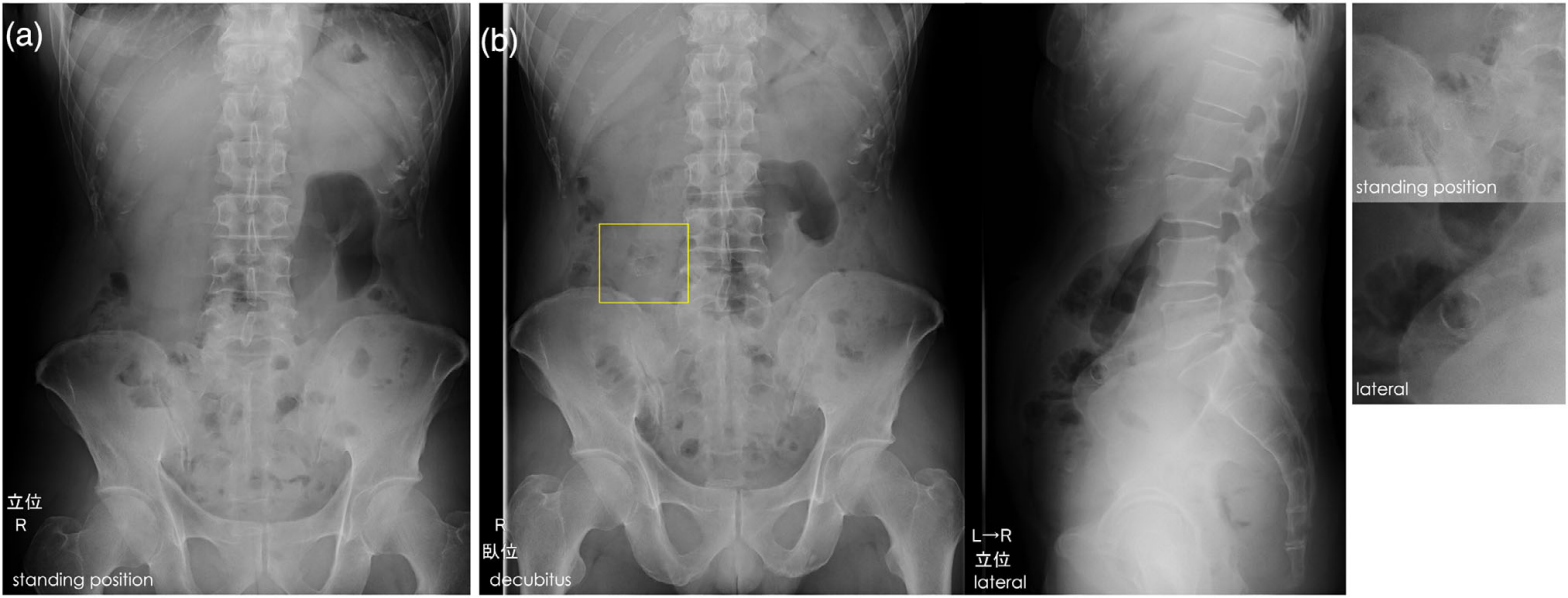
Plain abdominal X‐ray imaging. (a) Plain abdominal radiography (standing position) showed no patency capsule or shape deformity. (b) Plain abdominal radiographs (lateral and decubitus positions) showing the localization of the patency capsule (PC, in the lateral and decubitus views. The PC had already dissolved into a shell only (yellow box).
